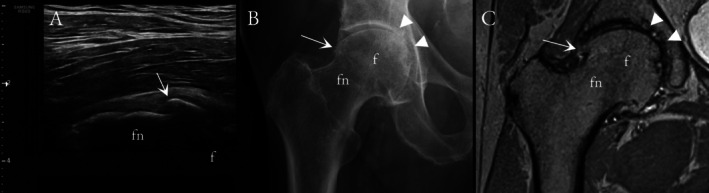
A 69‐year‐old male with a moderate‐sized femoral neck osteophyte (white arrow) visualized in longitudinal US (A), anteroposterior CR (B), and coronal PD‐weighted MRI plane (C). The osteophyte is well delineated in both US and CR. Additional medial joint space narrowing and subchondral bone changes (arrowheads) are visible in CR and MR, but not reachable by US. f, femoral head; fn, femoral neck.
